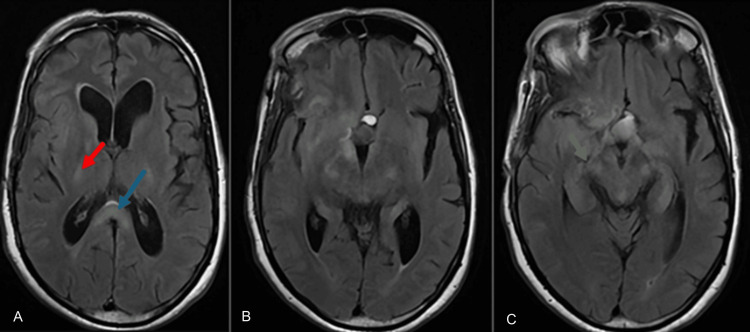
Axial T2-FLAIR MRI findings Axial T2-FLAIR MRI demonstrates hyperintensity along the pyramidal tracts. A: in the posterior limb of the internal capsule (red arrow) and in the splenium of the corpus callosum (blue arrow); B: showing the downward continuity of the signal abnormality from the internal capsule; C: extending further into the pontine region (green arrow). FLAIR: fluid attenuated inversion recovery
